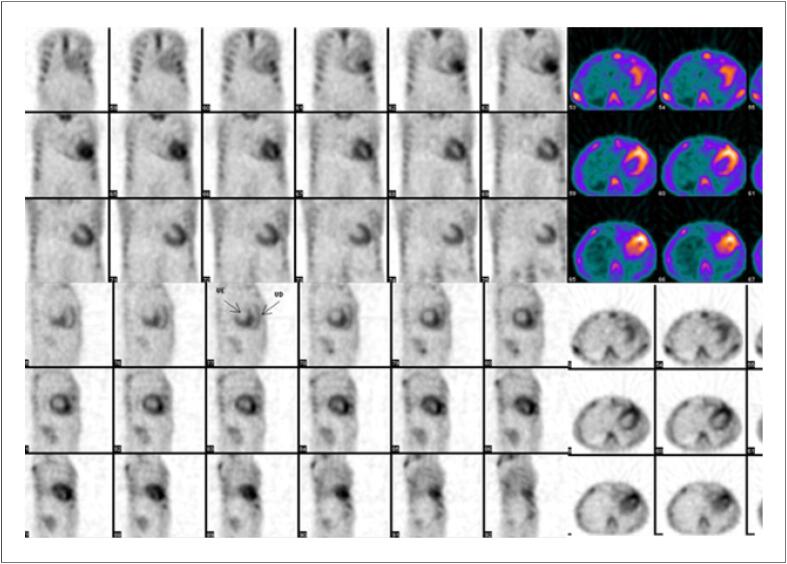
Imagens tomográficas (SPECT) reconstruídas nos eixos axial, coronal e sagital, identificando concentração do pirofosfato^99m^Tc no ventrículo esquerdo e acometimento simultâneo do ventrículo direito (setas) em paciente com suspeita de amiloidose cardíaca forma transtirretina (TTR) mutada, confirmada por teste genético.
